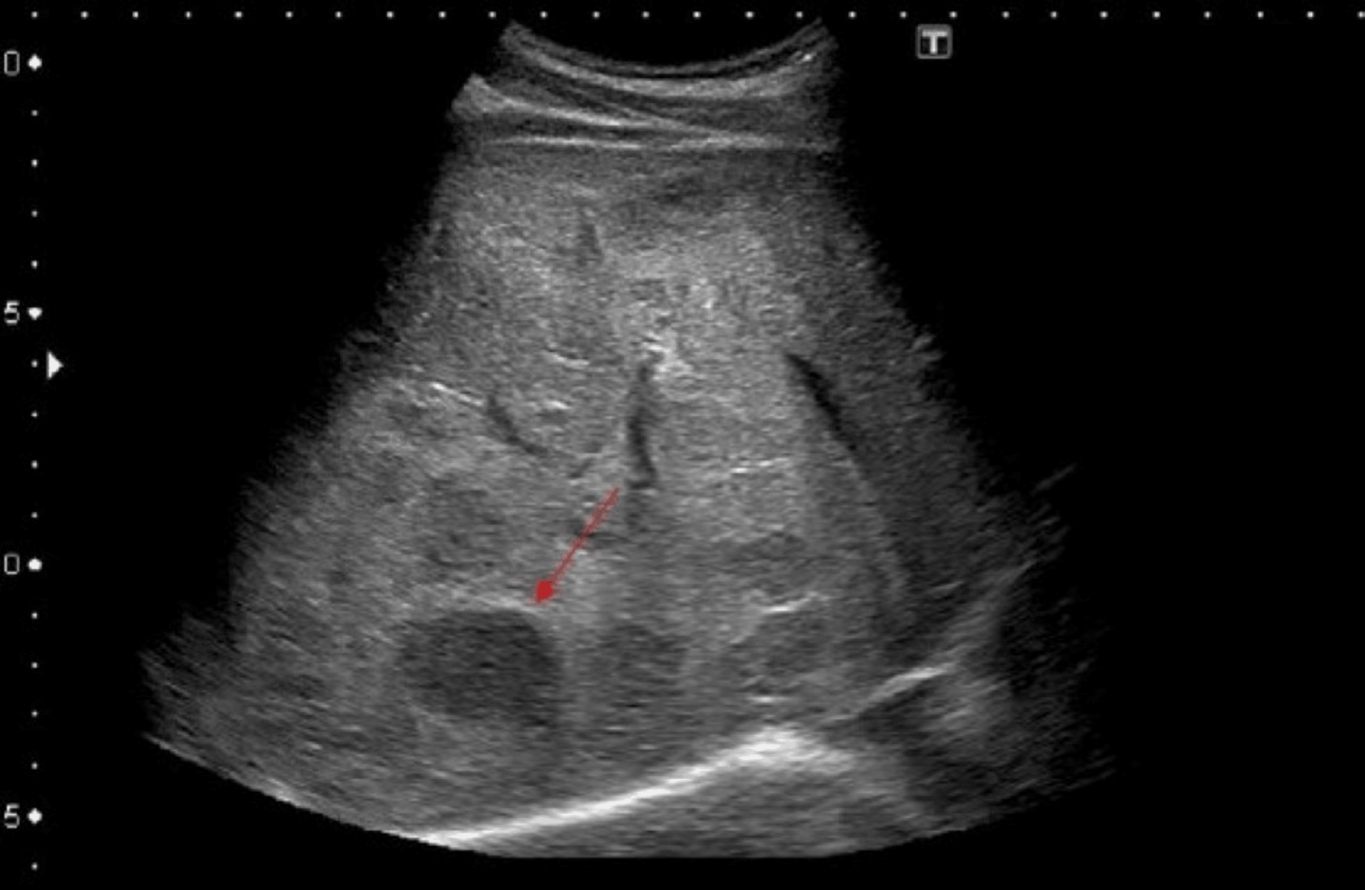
Ultrasound of the liver showing a large, well-defined, heterogenous lesion with central necrotic areas (red arrow), with the rest of the liver parenchyma of normal echotexture
